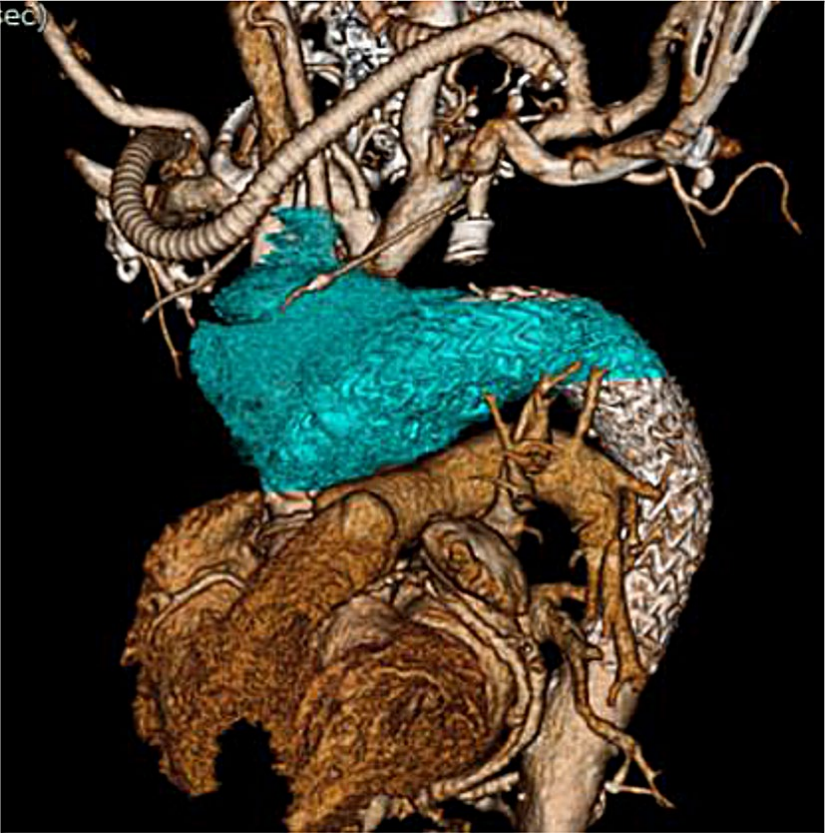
The CT value of peri-graft fluid collection (Blue color) was 30–40 HU
